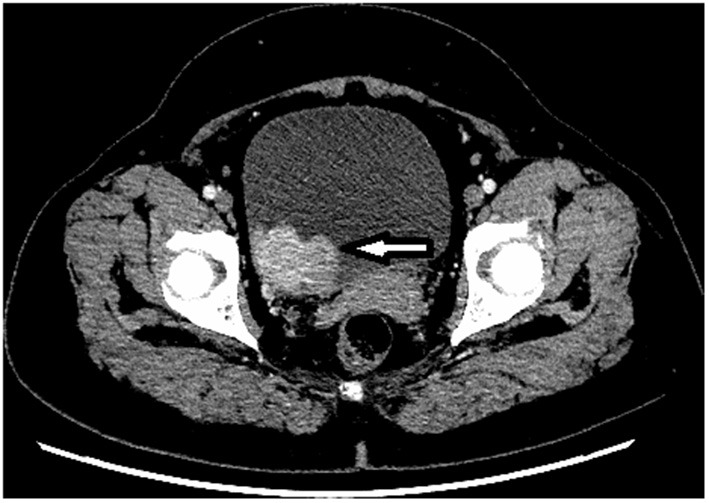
CT enhancement showing the lesion in the right wall of the urinary bladder.
